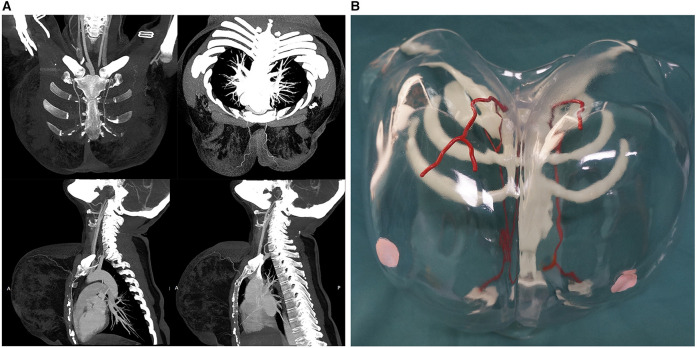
(A) Traditional computed tomography angiography. (B) Innovative three-dimensional (3D)-printed breast vascular model. The individualized 3D-printed breast vascular model includes the dominant supplying arteries (red), transparent chest with marked nipples (pink), sternum (white), and partial ribs (white).
